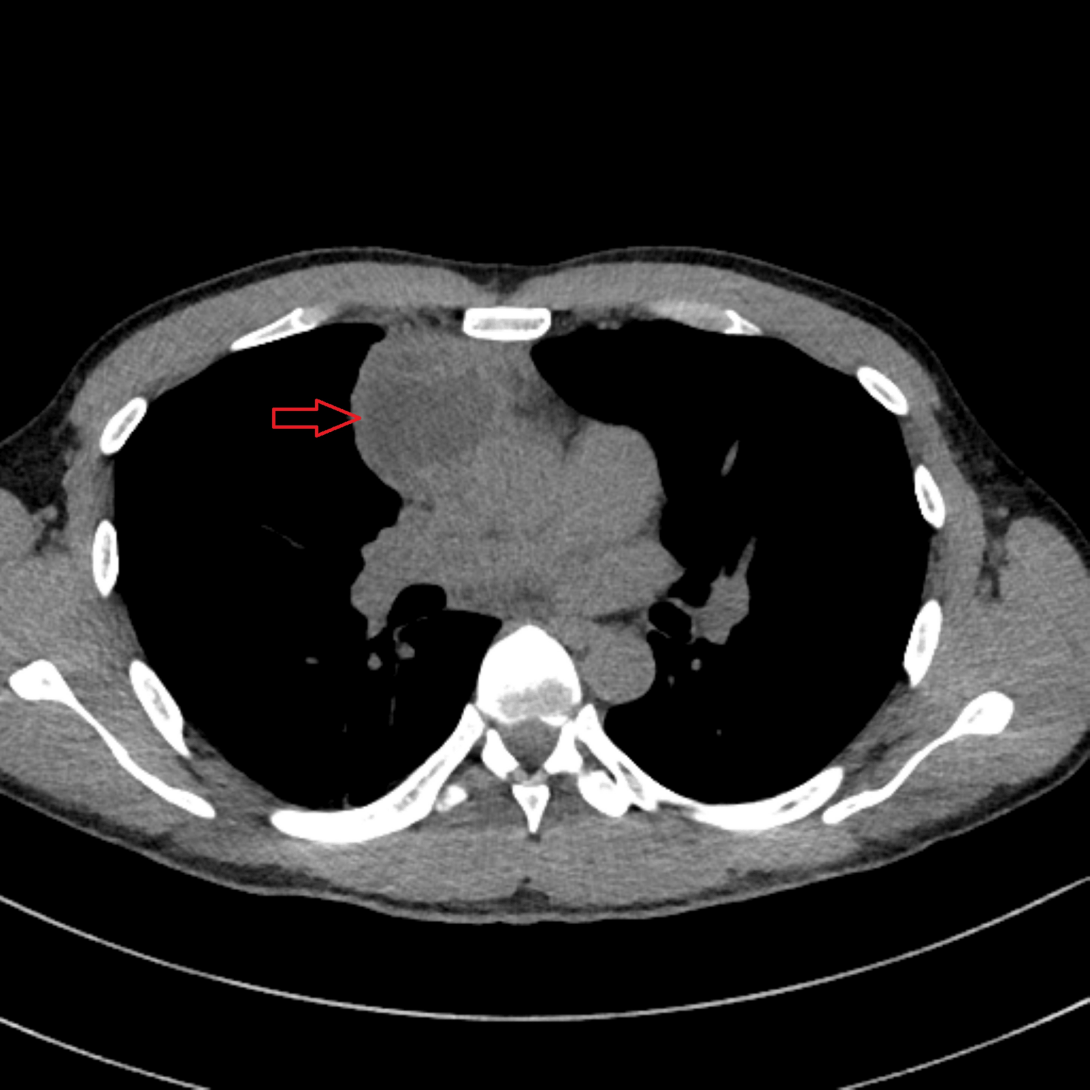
Axial CT scan image exhibiting an anterior mediastinal mass, with central areas of liquid density, and its relationship with surrounding structures
